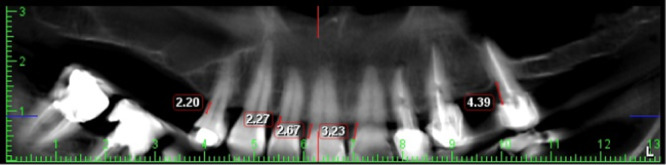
Measuring bone loss in panoramic view of CBCT images.
